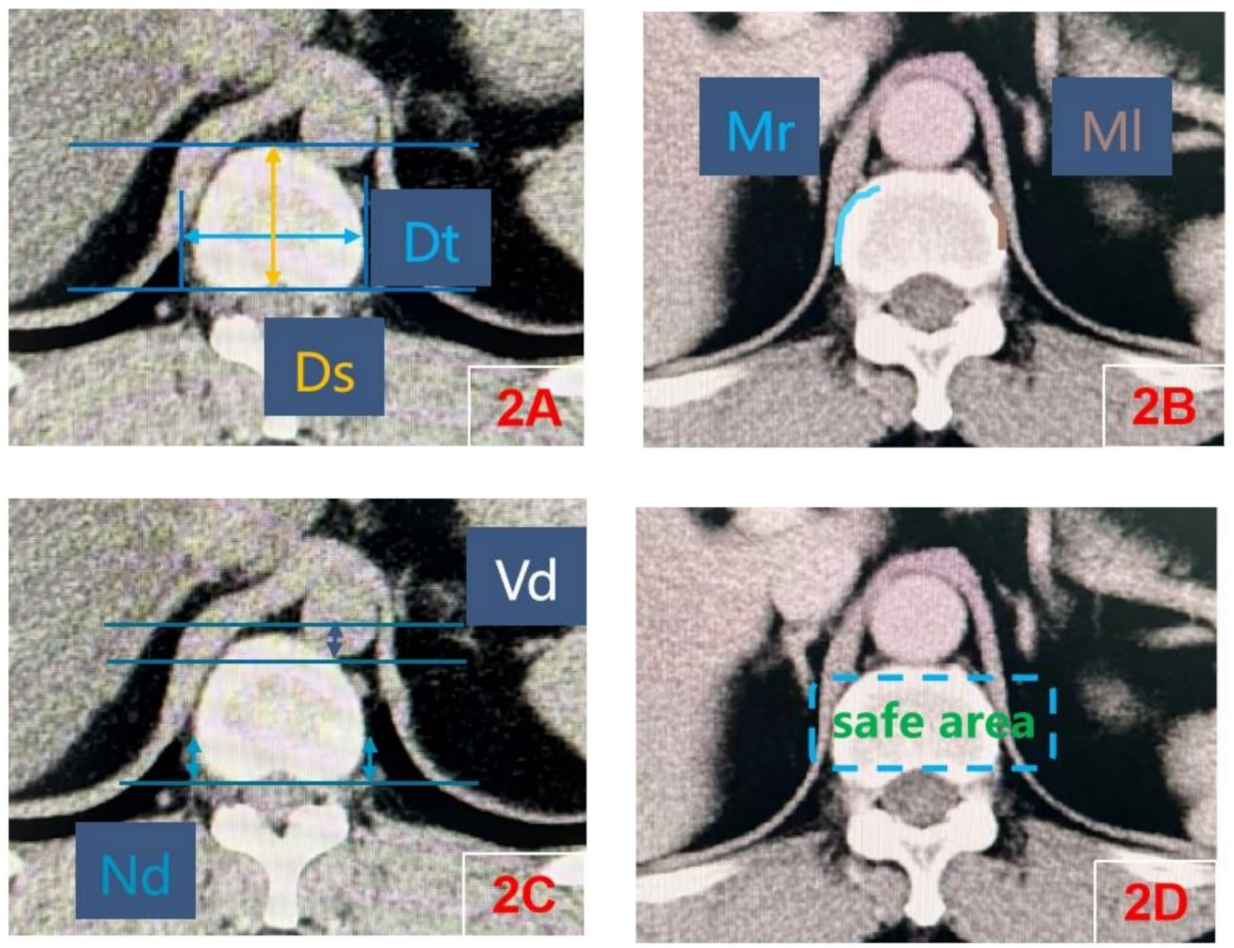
CT measurements: A, Measurement of vertebral endplate diameter(Dt transversal diameter, Ds sagittal diameter); B, Measurement of muscle attachment area(Mr the right muscle attachment area, Ml the left muscle attachment area); C, Measurement of vascular position(Vd) and nerve root position(Nd); D, Measurement of safe area
